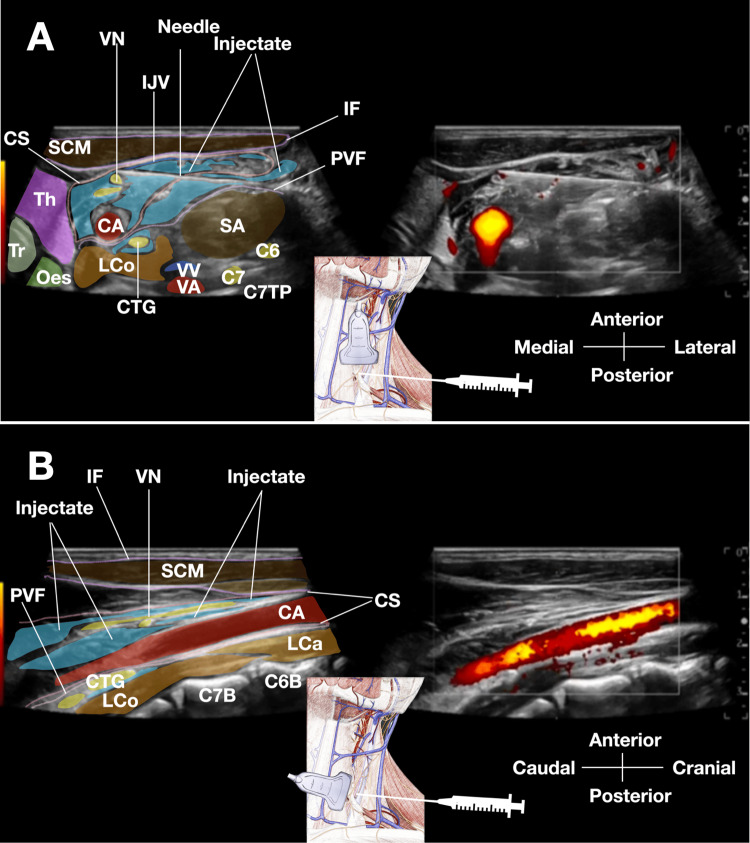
Hydrodissection of the vagus nerve within the carotid sheath. (A) Transverse ultrasound view at the C7 level during vagus nerve hydrodissection. The color-labeled sonogram demonstrates hydrodissection of the VN within the carotid sheath after sympathetic chain hydrodissection. The needle has been redirected to enter the CS. Slow 5% dextrose injection in water (anechoic fluid, colored in light blue) creates a 360° fluid halo completely surrounding the vagus nerve, separating it from the posteromedial wall of the common CA and surrounding fascial attachments. Of note, the characteristic compression and separation of the IJV from the carotid artery occurs as the injectate accumulates within the sheath. The previously hydrodissected CSC is visible posterior to the prevertebral fascia. (B) A sagittal ultrasound view of the vagus nerve within the carotid sheath. The color-labeled sagittal sonogram demonstrates the longitudinal view of the VN during hydrodissection. The transducer has been rotated 90° to obtain this sagittal plane through the carotid sheath. The needle is positioned within the sheath (not shown), and the injected 5% dextrose in water (bluish coloration) surrounds the vagus nerve along its longitudinal axis, confirming complete perineural hydrodissection. The vagus nerve is visualized as a hypoechoic tubular structure with hyperechoic borders, now separated from the surrounding fascial planes by the injectate. The common CA is seen in its longitudinal orientation. C7: C7 nerve root; C7B: C7 vertebral body; C7PT: C7 posterior tubercle; CA: common carotid artery; CSC: cervical sympathetic chain; CS: carotid sheath; IJV: internal jugular vein; IF: investing fascia of sternocleidomastoid; LCa: longus capitis; LCo: longus colli; Oes: esophagus; OMH: omohyoid; SA: scalenus anterior; SCM: sternocleidomastoid; SM: scalenus medius; SP: scalenus posterior; Th: thyroid; Tr: trachea; VA: vertebral artery; VN: vagus nerve; VV: vertebral vein The color shading and labeling were created in Keynote by Professor King-Hei Stanley Lam.
